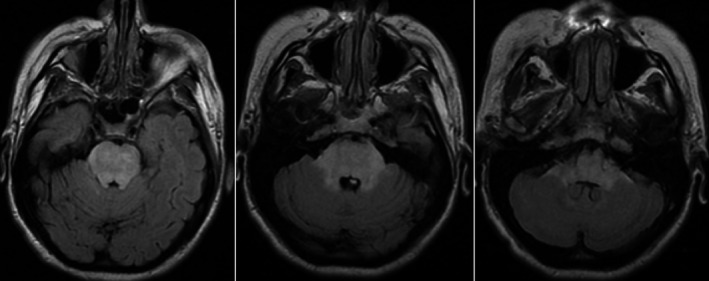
FLAIR MRI on day 1 of admission. Note hyperintense signaling in the entirety of the pons, and partial cerebellum
